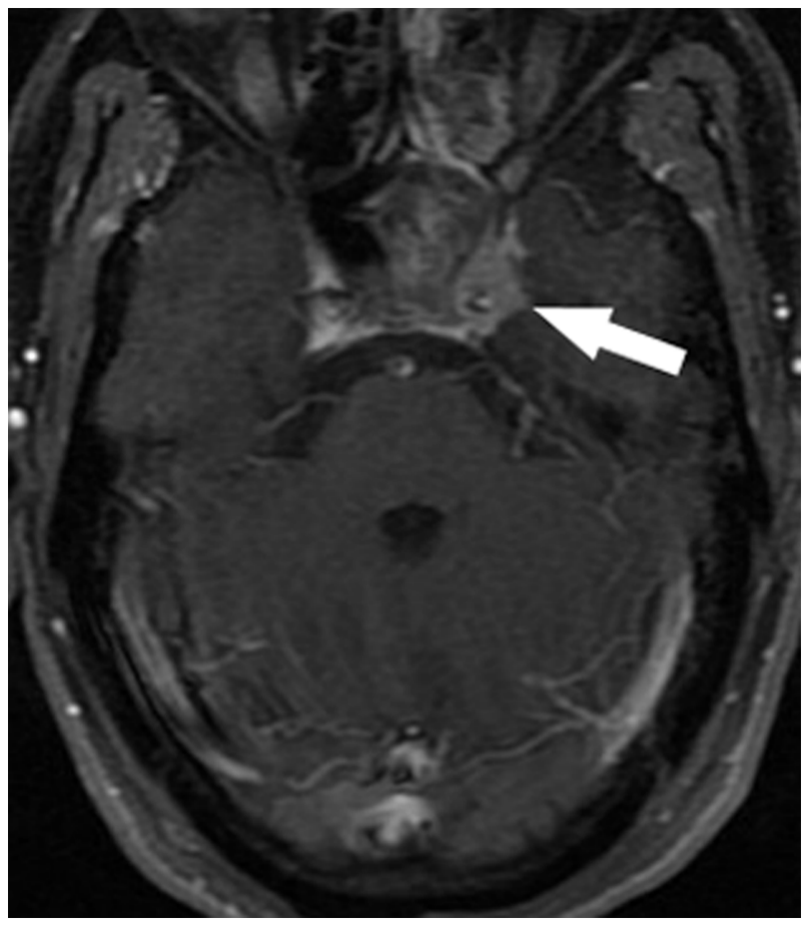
42-year-old man with nasopharyngeal carcinoma with cranial nerve invasion: enhanced axial T1-weighted image with fat suppression shows tumor invasion in the left cavernous sinus (arrow).
